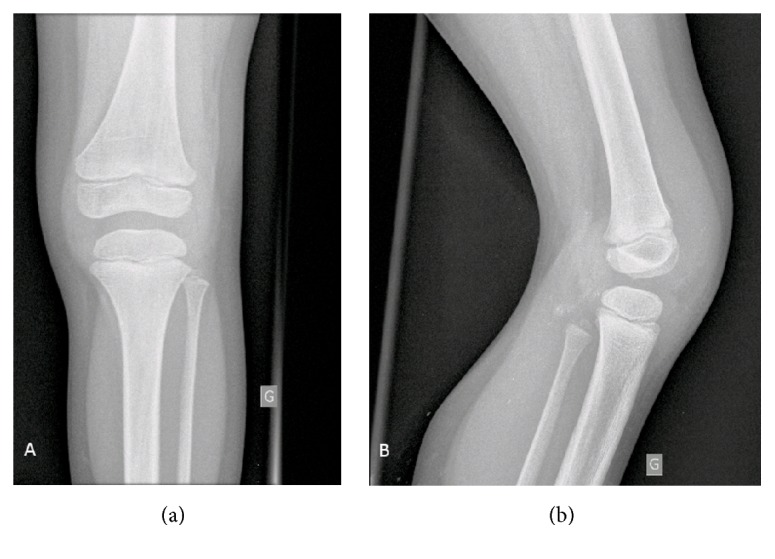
Plain, anteroposterior (a) and lateral (b) radiographs of a 6-year-old male patient's left knee showing hypoplasia of the tibial intercondylar eminence and of the femoral intercondylar notch.
